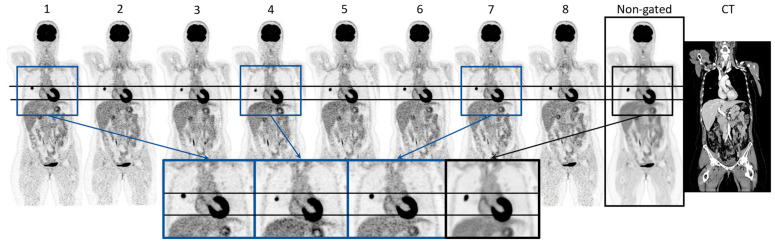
Visualization of the respiratory gated reconstruction (gate 1–8) containing, along with the non-gated reconstruction image and CT of patient 16. The horizontal lines indicate the top position of the tumor and the liver referenced at gate 1. The zoomed images of gates 1, 4, and 7, and the non-gated image highlight the differences in the position and appearance of the tumor and the liver.
